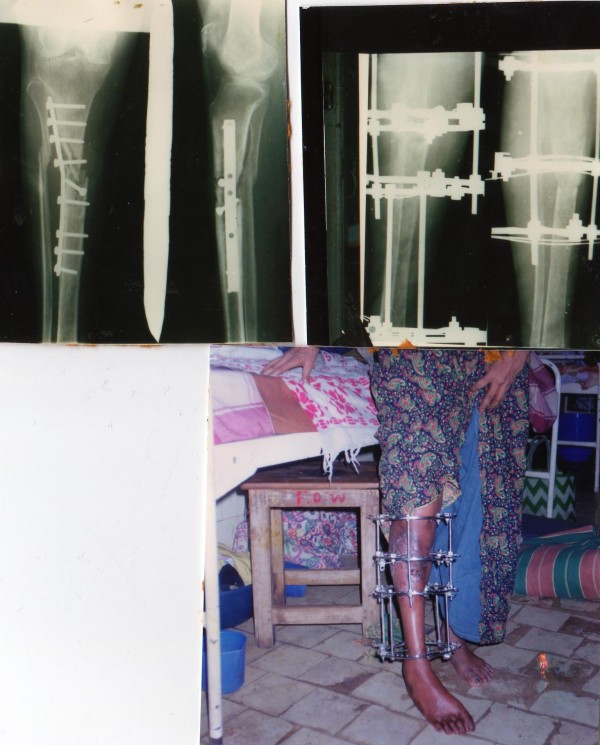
Patient no 3: (clockwise from top left).  Pre-operative x-ray of the tibia; with fixator in situ at 100 days: and at 6 months.
